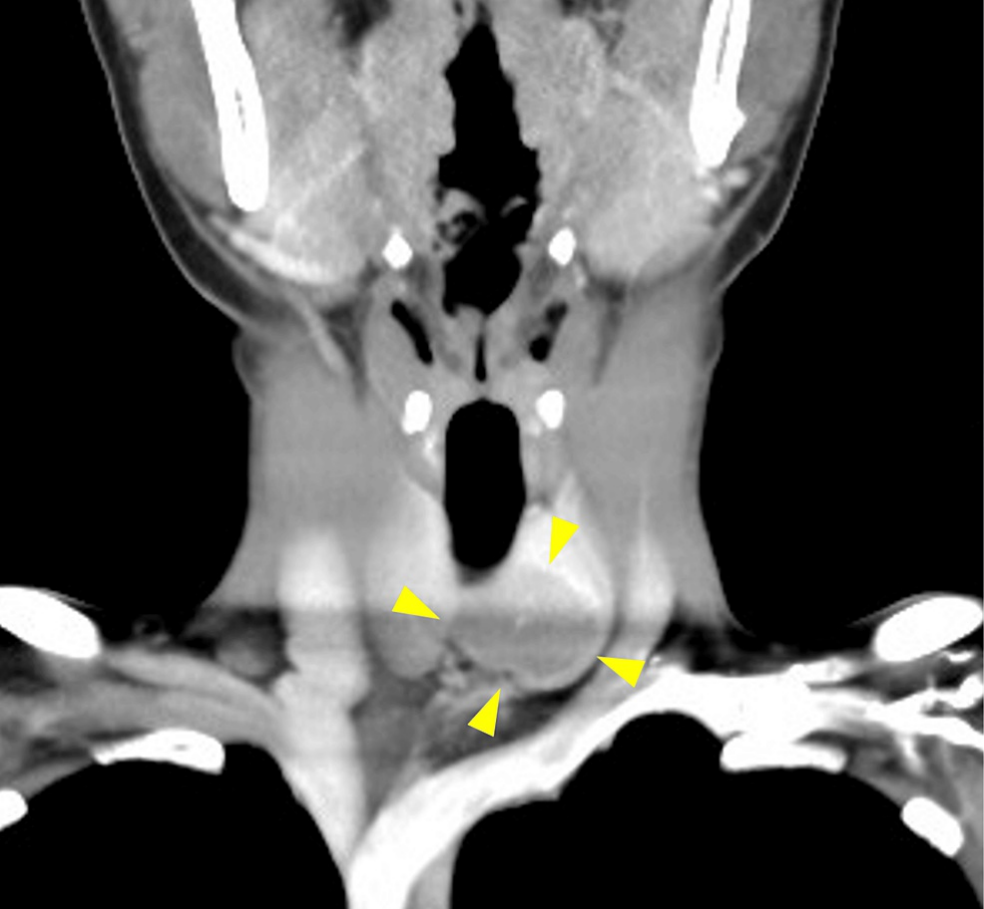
CT (coronal) A low-density area from the left lobe to the isthmus CT: computed tomography
